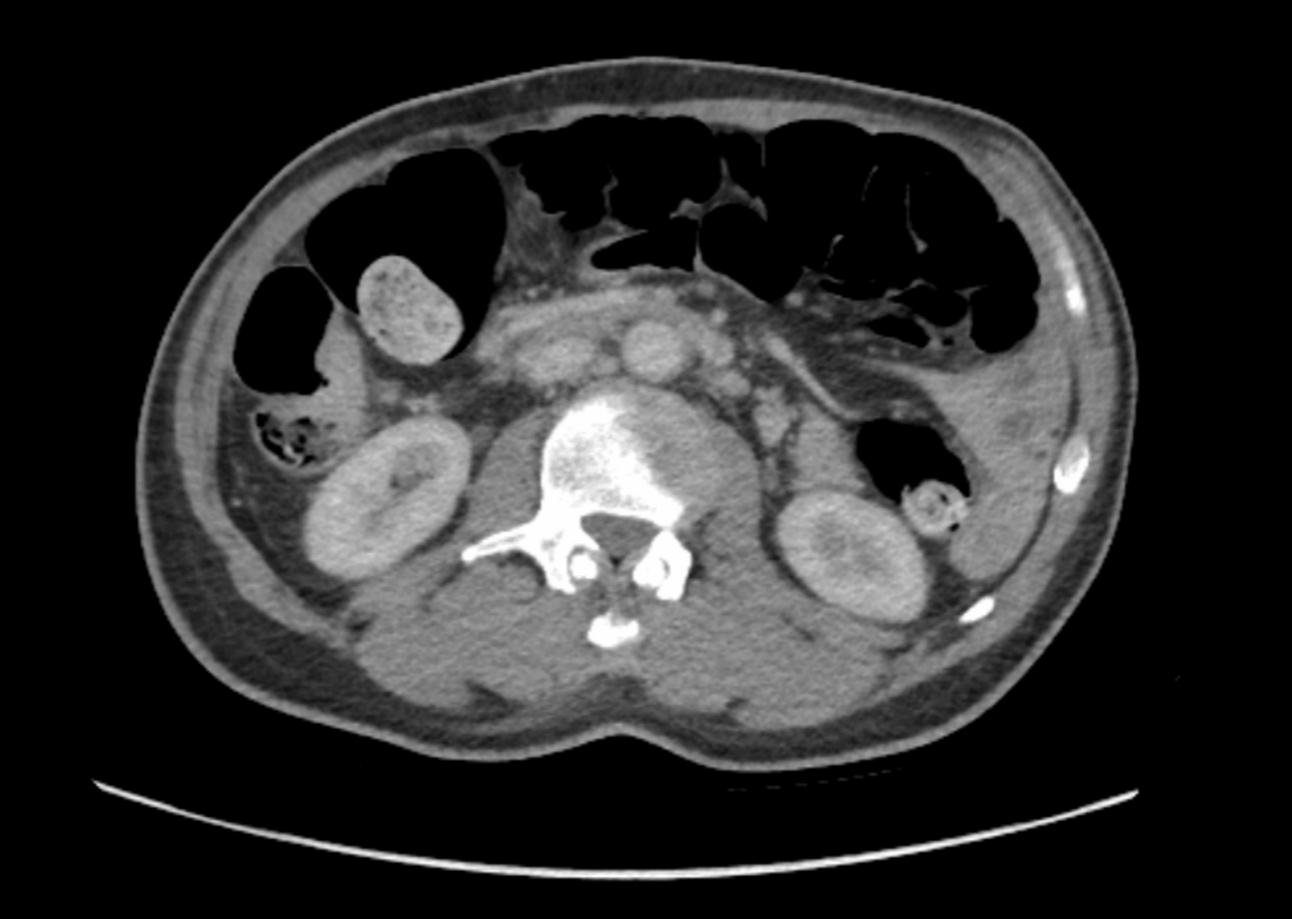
Abdominal CT showing focal wall thickening of the colon
